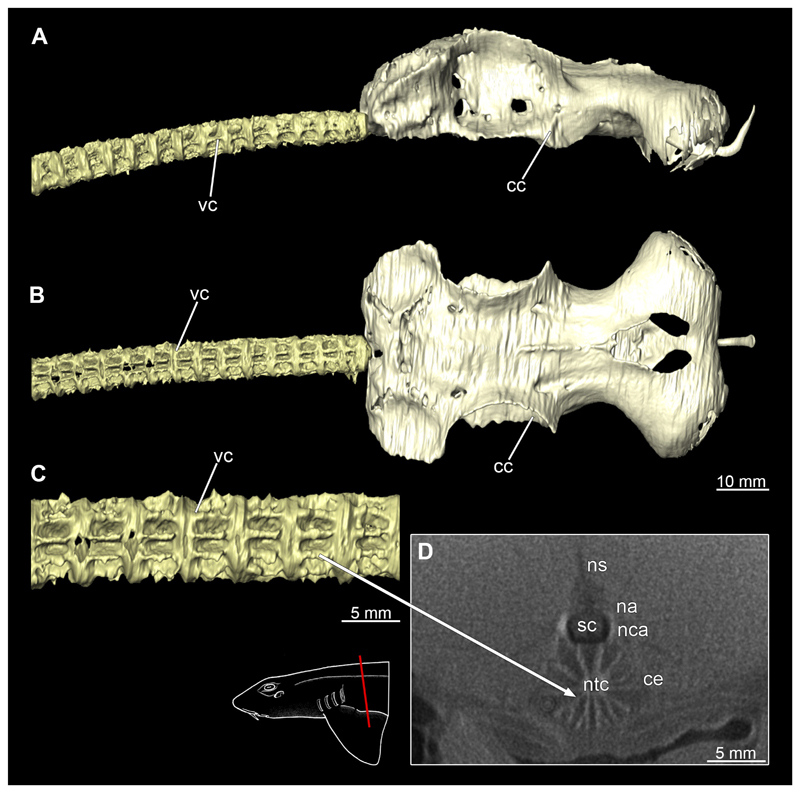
Chiloscyllium punctatum*, male: Vertebral column with chondrocranium as rendered surface. Arrow pointing to the longitudinal ribs running around the centre in the CT-image. A lateral, B dorsal, C detail lateral and D transversal cross-section through dorsal region (CT-image). Abbreviations: cc, chondrocranium; ce, centrum; na, neural arch; nca, neural canal; ns, neural spine; ntc, notochord; sc, spinal cord; vc, vertebral column.
